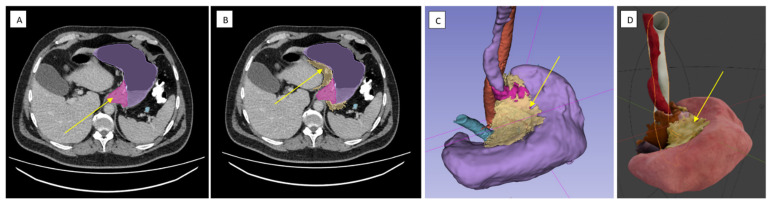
Stages of 3D reconstruction. (A) Abdominal CT scan with demarcation of gastric tumor (arrow) and unaffected stomach tissue; (B) abdominal CT scan showing annotated mesogastric layer (arrow); (C) 3D reconstruction of mesogastric layer (arrow) and tumor using 3D Slicer software; (D) volume-rendered (VR) reconstruction model (mesogastric layer is indicated with arrow).
